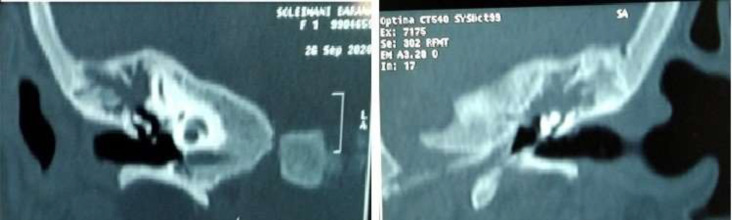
The High Resolution computed tomography (HRCT) of temporal showed bilateral effusion of middle ear
